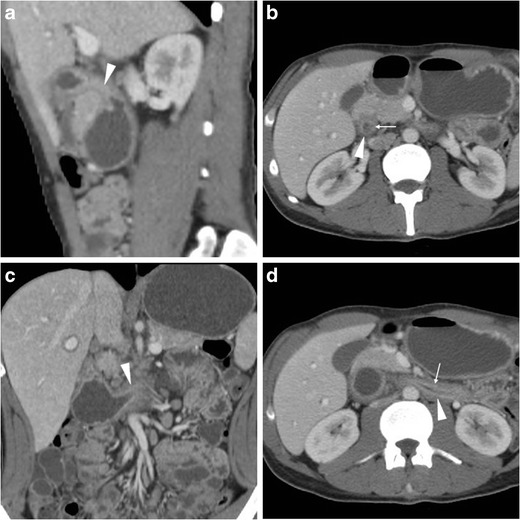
A 31-year-old male with history of ileocecal resection for Crohn’s disease (CD) seven years earlier underwent CT-enterography with oral polyethylene glycol solution: the endoscopically diagnosed impassable stricture was well depicted between first and second duodenum (arrowheads in a, b), and a second, longer cd lesion was seen the fourth duodenum (arrowheads in c, d). Both strictures showed characteristic mural stratification and enhancing mucosa (thin arrows) of active CD
